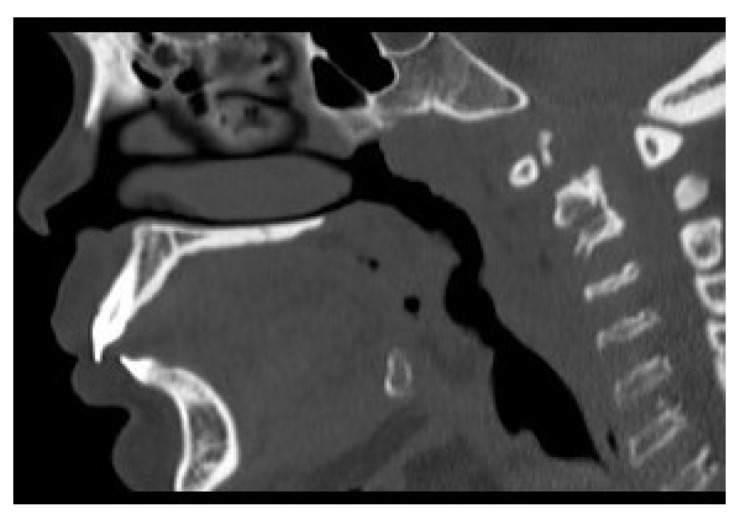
CT scan of MPS IV showing contracted nasopharynx, large tongue, prominent teeth, and high larynx.
